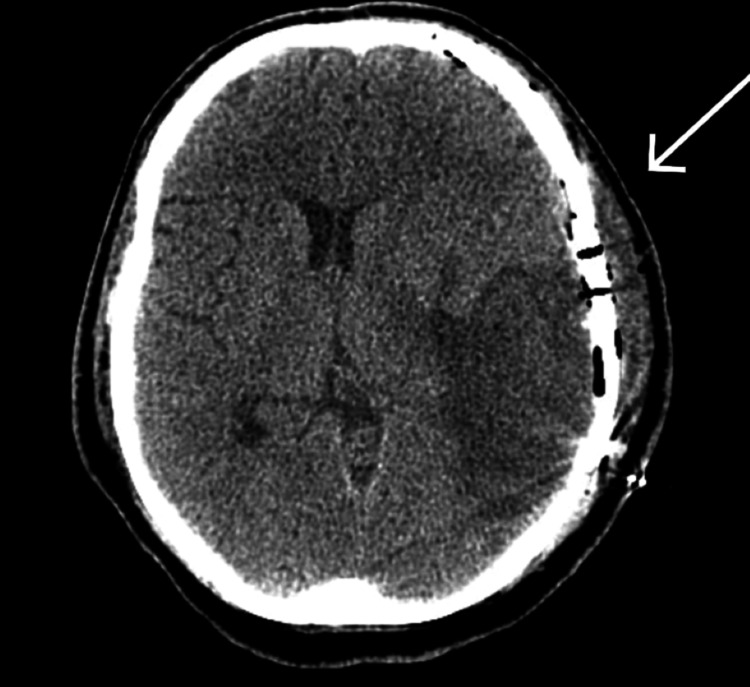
Post-cranioplasty CT brain, with the arrow representing distinct improvements of the lesion, and a decreased midline shift in the image is also appreciable.
